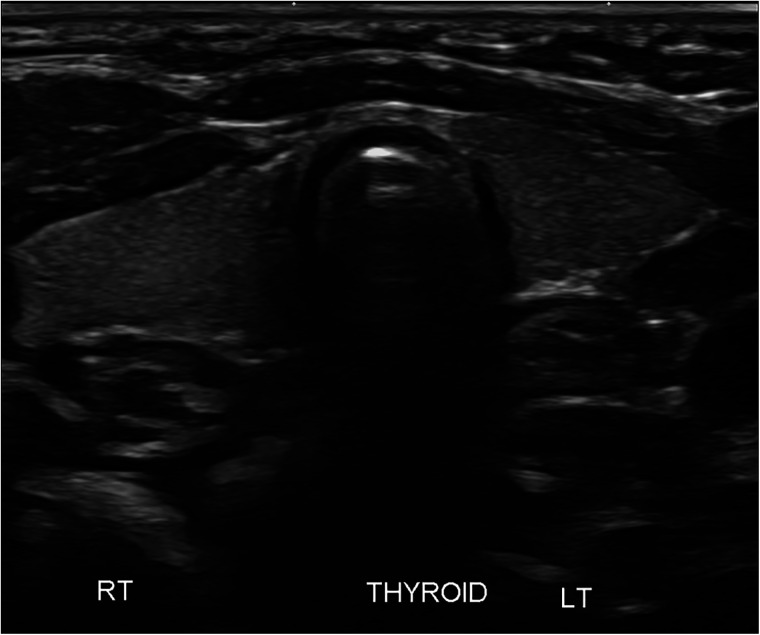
Ultrasound of the neck showing clear presence of the thyroid gland in its normal anatomical location.
